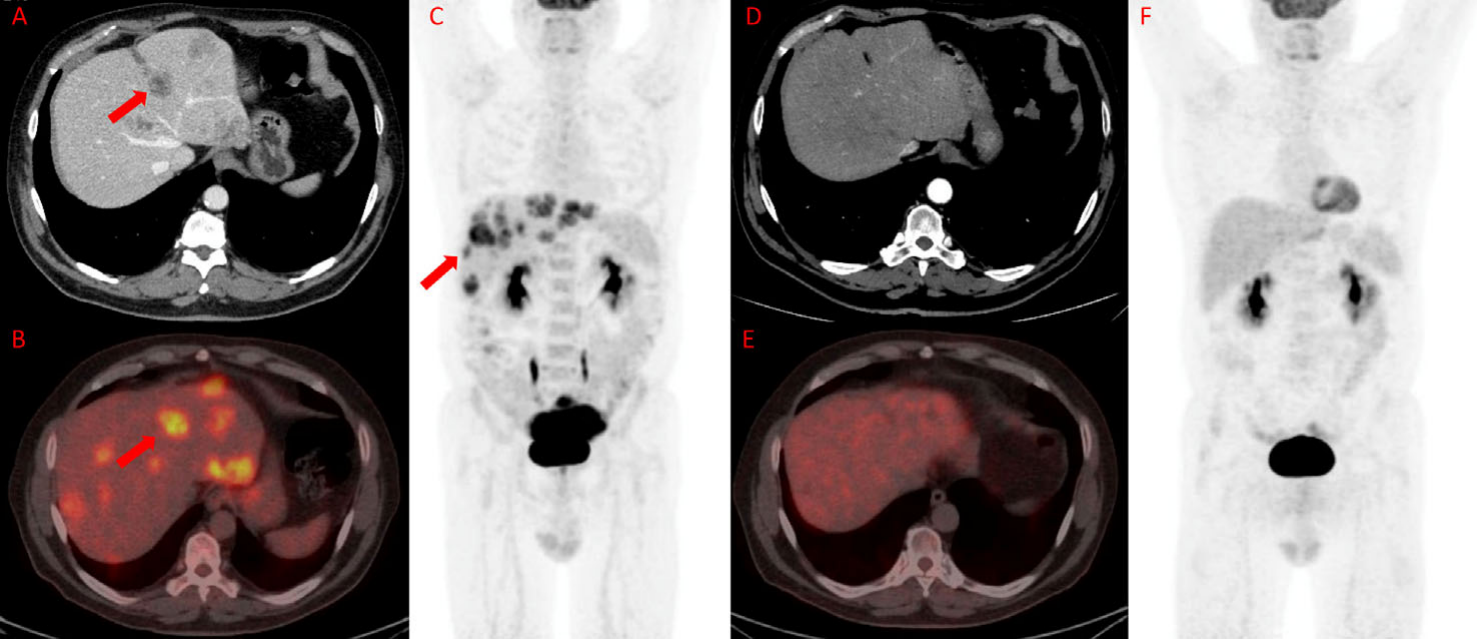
^18^F-FDG PET/TC in a patient affected by colon cancer with liver metastasis. A 55-year-old male affected by colorectal cancer with multiple liver metastases, subjected to 12 cycles of Bevacizumab-based chemotherapy and 24 hyperthermia sessions on the abdomen as first-line. Baseline ceCT showed massive liver involvement (A and red arrows) and whole-body ^18^F-FDG PET/TC showed increased ^18^F-FDG uptake in the liver lesions (red arrows) detectable also on axial fused PET/CT and MIP images (B and C) in the same site. Timepoint-2 ceCT (D) evaluation demonstrated significant size decrease of liver metastasis with no evidence of ^18^F-FDG uptake on whole-body PET/CT (E and F). According to RECIST, the patient was classified as CR.
